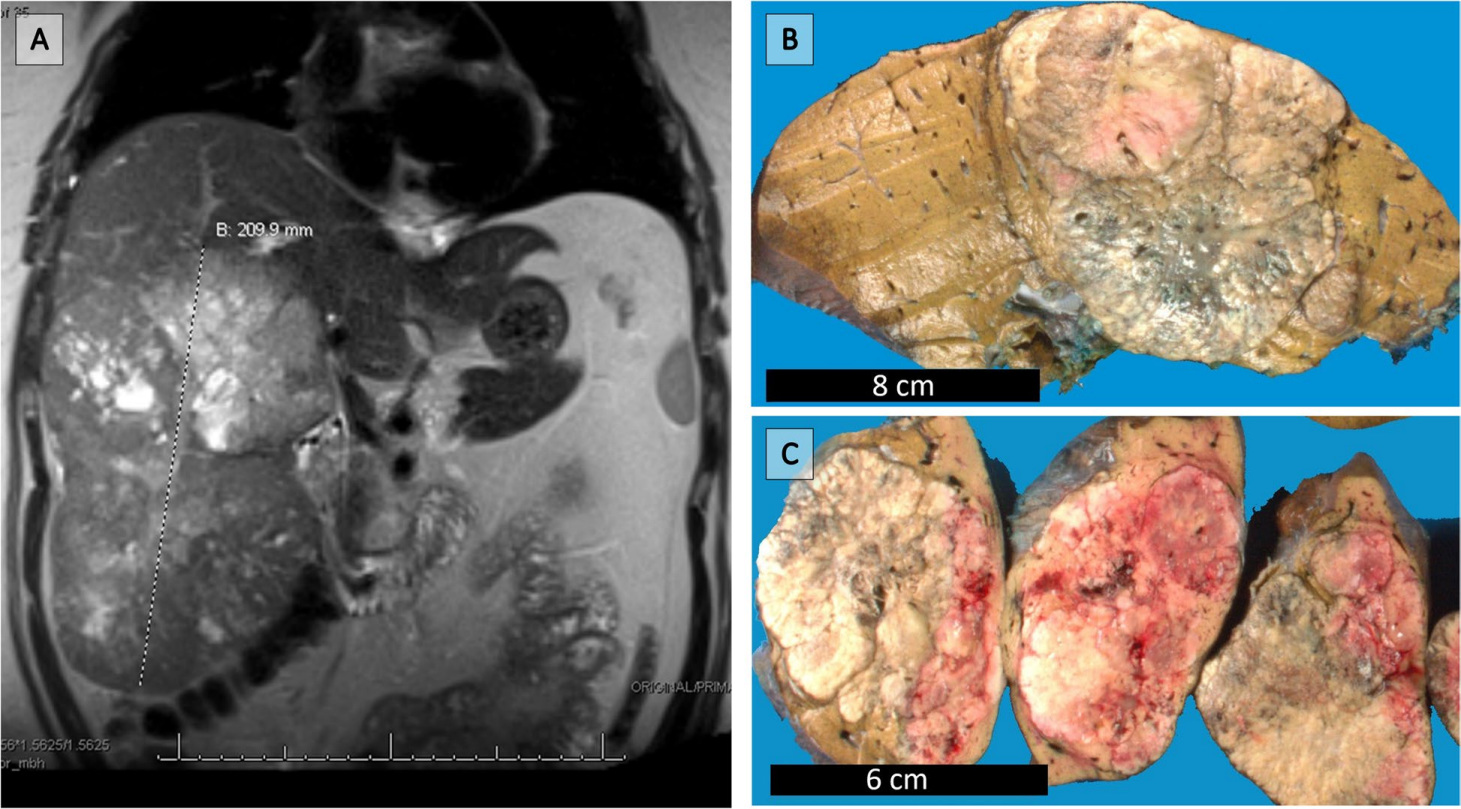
T2 MRI shows an enlarged liver measuring 29.0 × 22.0 x 10.0 cm with mass lesion (A). Grossly identified are two tan-white to tan-pink, focally hemorrhagic abutting tumors measuring 21.5 cm and 4.5 cm (B and C)
